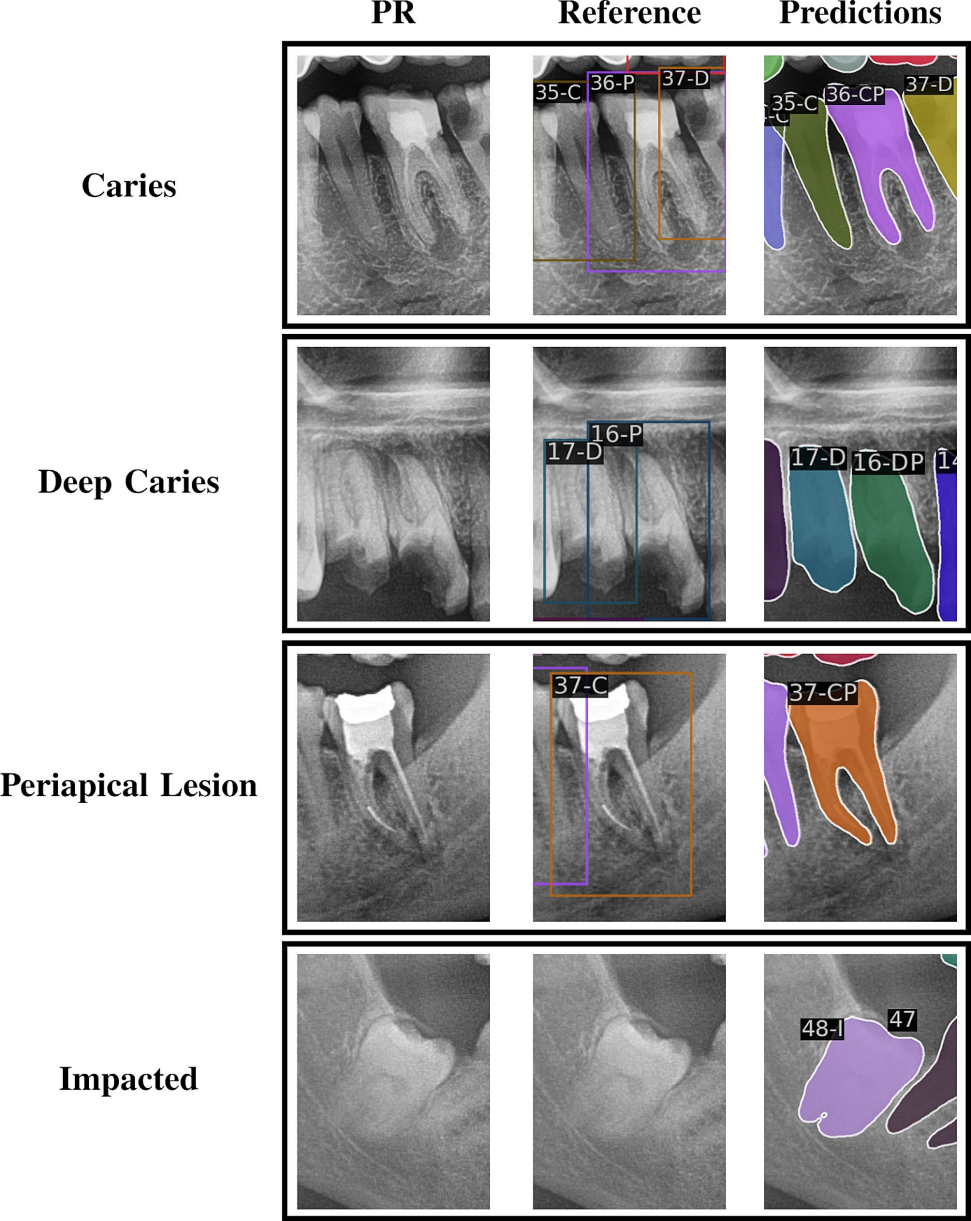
DENTEX annotations with missing diagnoses. A missed diagnosis (false negative) is shown for each diagnosis, which are selected based on the maximum difference between a diagnosis probability and whether that diagnosis is annotated. Note that the DENTEX dataset only annotates diagnosed teeth, whereas the current method predicts all teeth. The label is the tooth’s FDI number with C = early caries, D = deep caries, P = periapical lesion, I = impacted
